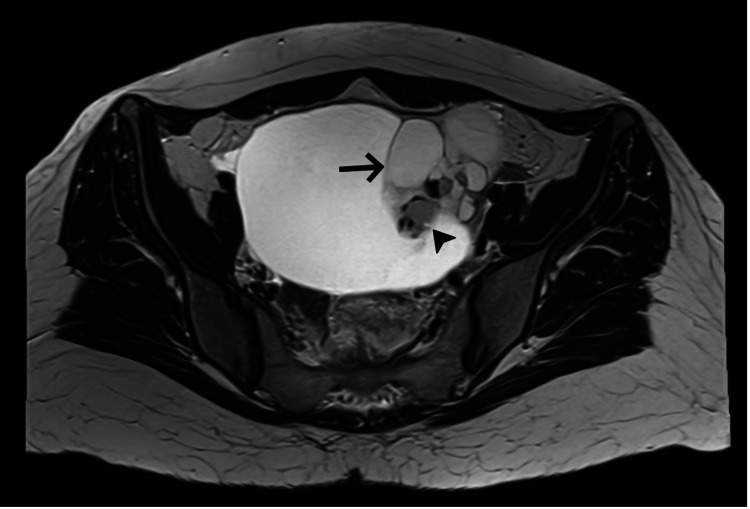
Axial T2-weighted MRI showing a benign-appearing multiloculated ovarian cyst mimicking an epithelial neoplasm. Axial pelvic MRI demonstrating a large multiloculated left adnexal cystic lesion. An internal septation (arrow) and a mural solid component (arrowhead) are identified, contributing to the preoperative suspicion of mucinous cystadenoma.
